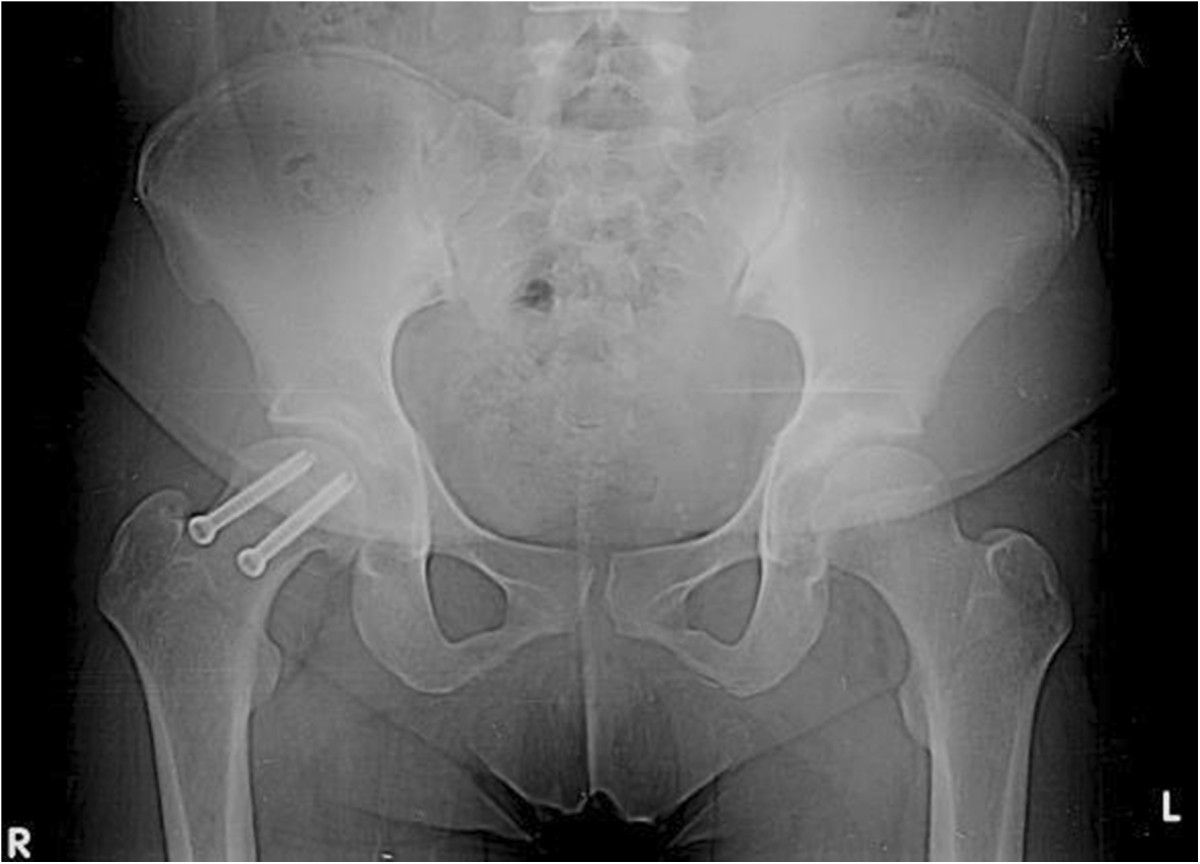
X-ray control. On this image obtained 12 months after treatment, appropriate situation of osteosynthetic material can be seen, in addition to reduced physis.
